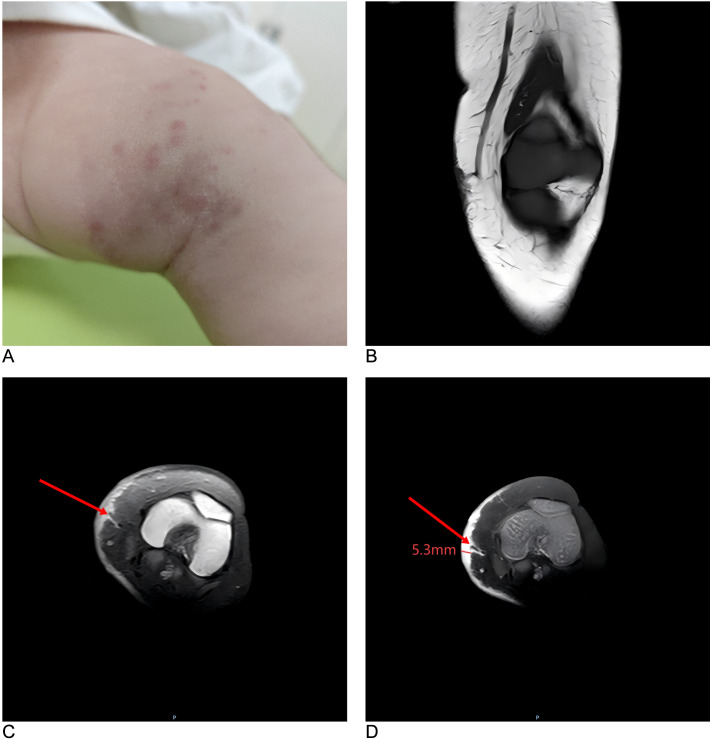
Clinical and MRI examination of the pediatric patient. (A) Clinical examination shows a cutaneous mass on the left knee, with multiple purplish-red papules distributed on the surface. (B, C) MRI reveals a poorly demarcated, patchy lesion in the medial epidermal and subcutaneous tissues of the left knee, demonstrating hypointensity on T1-weighted coronal image and hyperintensity on T2-weighted axial image. (D) On contrast-enhanced T1-weighted axial image, the lesion shows a marked homogeneous enhancement, with abundant vascular supply and drainage. The arrow points at a slightly enlarged arterial flow void. MRI, magnetic resonance images.
